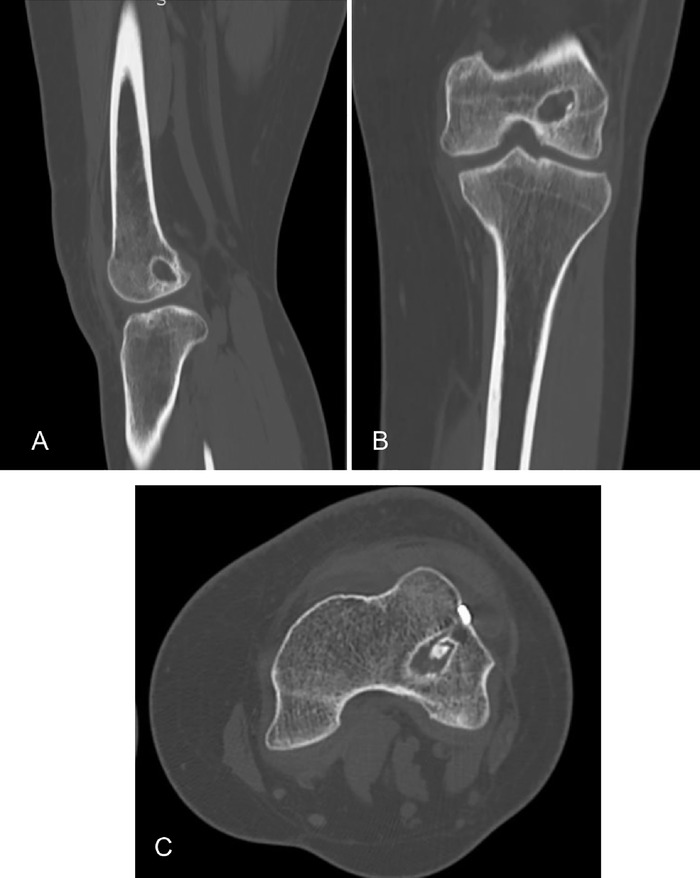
Computed tomography scans of the left knee show femur tunnel in (A) sagittal, (B) coronal, and (C) axial views.
